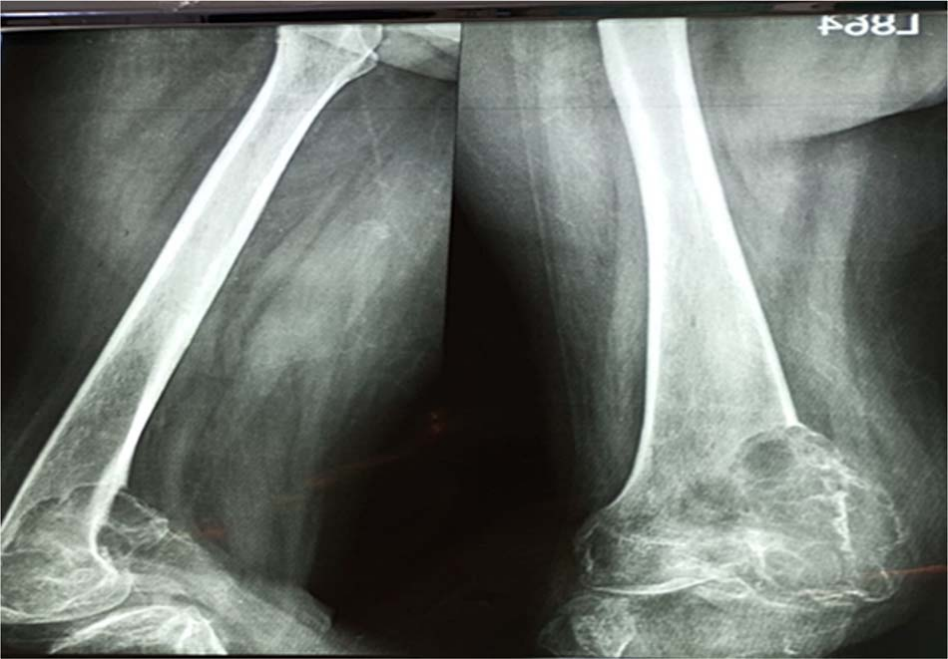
X-ray that was taken 4 months before presentation showing the tumor was very aggressive with a narrow zone of transition, and mass is progressively increased.
